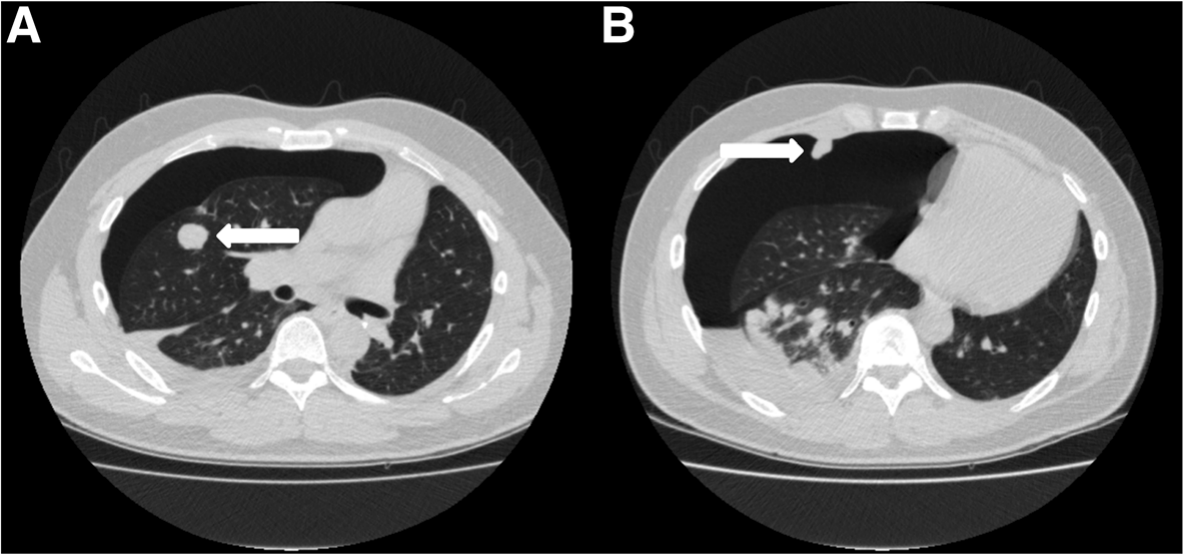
CT scans of patient 3. Routine follow-up CT scan showing the pneumothorax on the right side. A shows one of the pulmonary metastasis, B shows a pleural metastasis.
